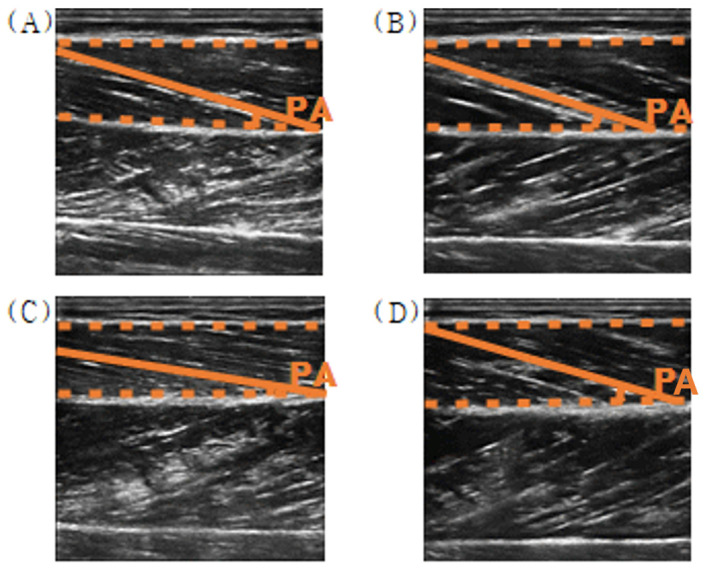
Example of the pennation angle in ultrasound images of the tibialis anterior muscle ((A) relaxed; (B) voluntary contraction, (C) involuntary contraction, and (D) eccentric contraction).
